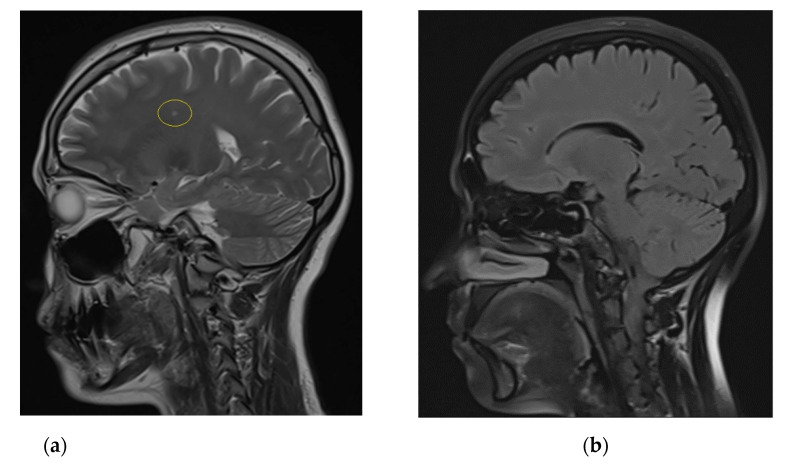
(a) Sagittal T2-weighted MRI scan of the brain shows active foci of demyelination in the left frontal lobe (yellow circle); (b) Sagittal T2 TIRM dark fluid MRI scan shows moderate tongue muscle atrophy.
